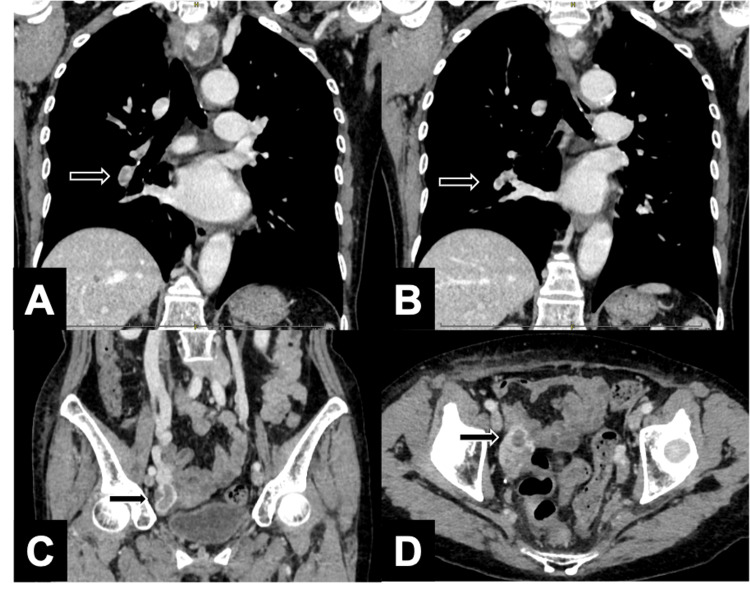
Postoperative contrast-enhanced CT findings A, B: Contrast-enhanced CT demonstrated filling defects in the pulmonary arteries (arrows), consistent with pulmonary thromboembolism (PTE). C, D: Postoperative CT revealed a thrombus in the right ovarian vein (arrows), consistent with ovarian vein thrombosis (OVT).
